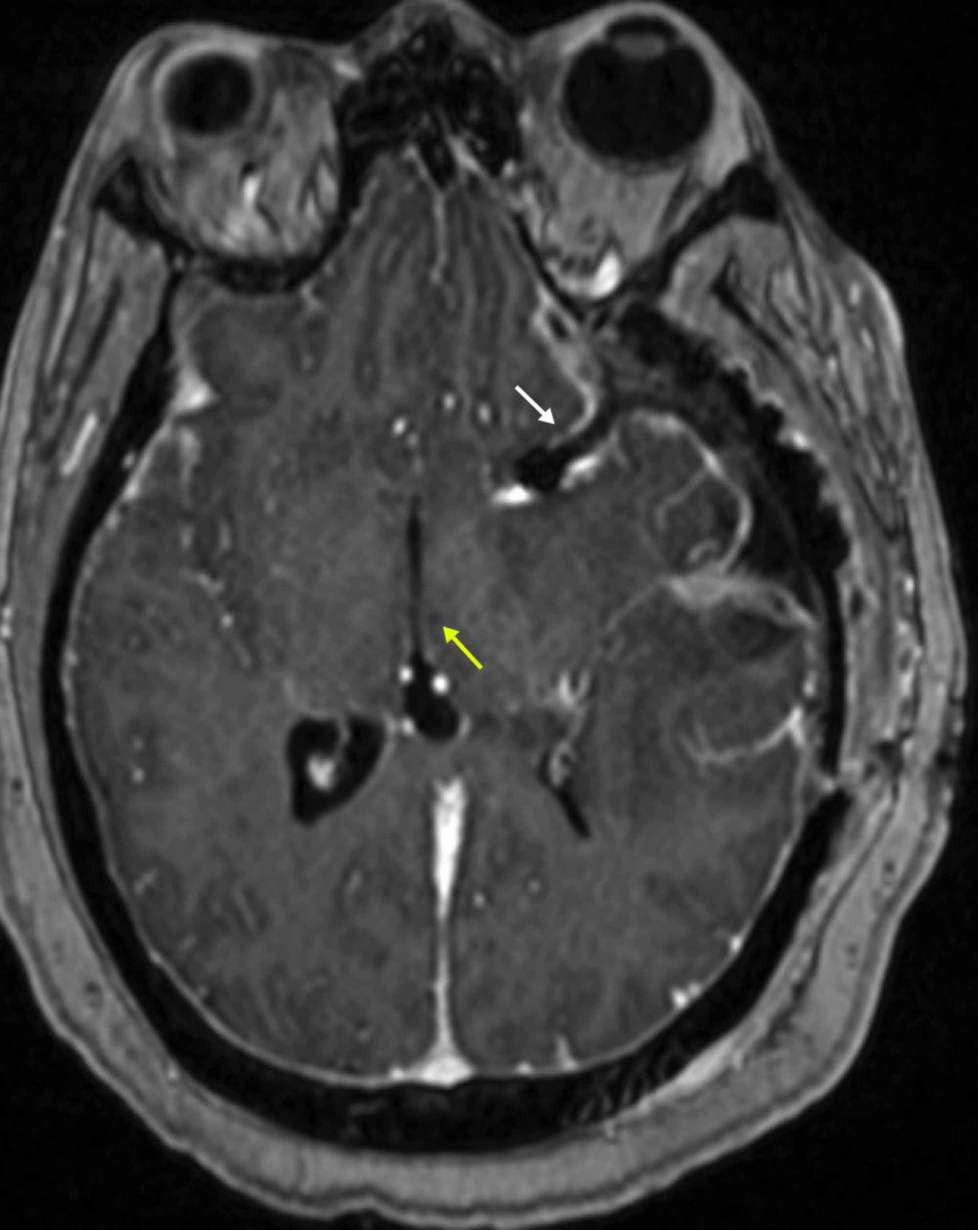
Postoperative MRI brain with contrast (axial view) shows the tumor resection bed (white arrow) and the thalamic punctate infarct (yellow arrow).
